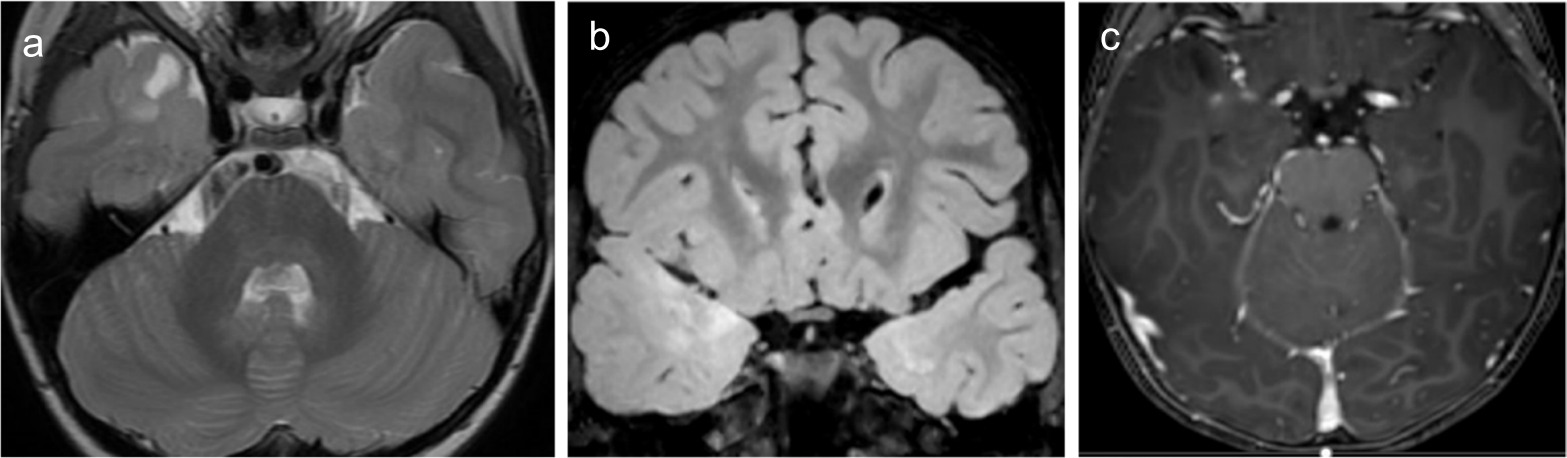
Diffuse low-grade glioma MAPK altered with *BRAF V600E* mutation in a 3-year-old male who presented with a seizure. Axial T2-weighted (a), coronal FLAIR (b), and axial T1-weighted contrast-enhanced (c) MR images demonstrate a T2/FLAIR hyperintense right temporal mass with indistinct posterior margins and a small nodular focus of enhancement
